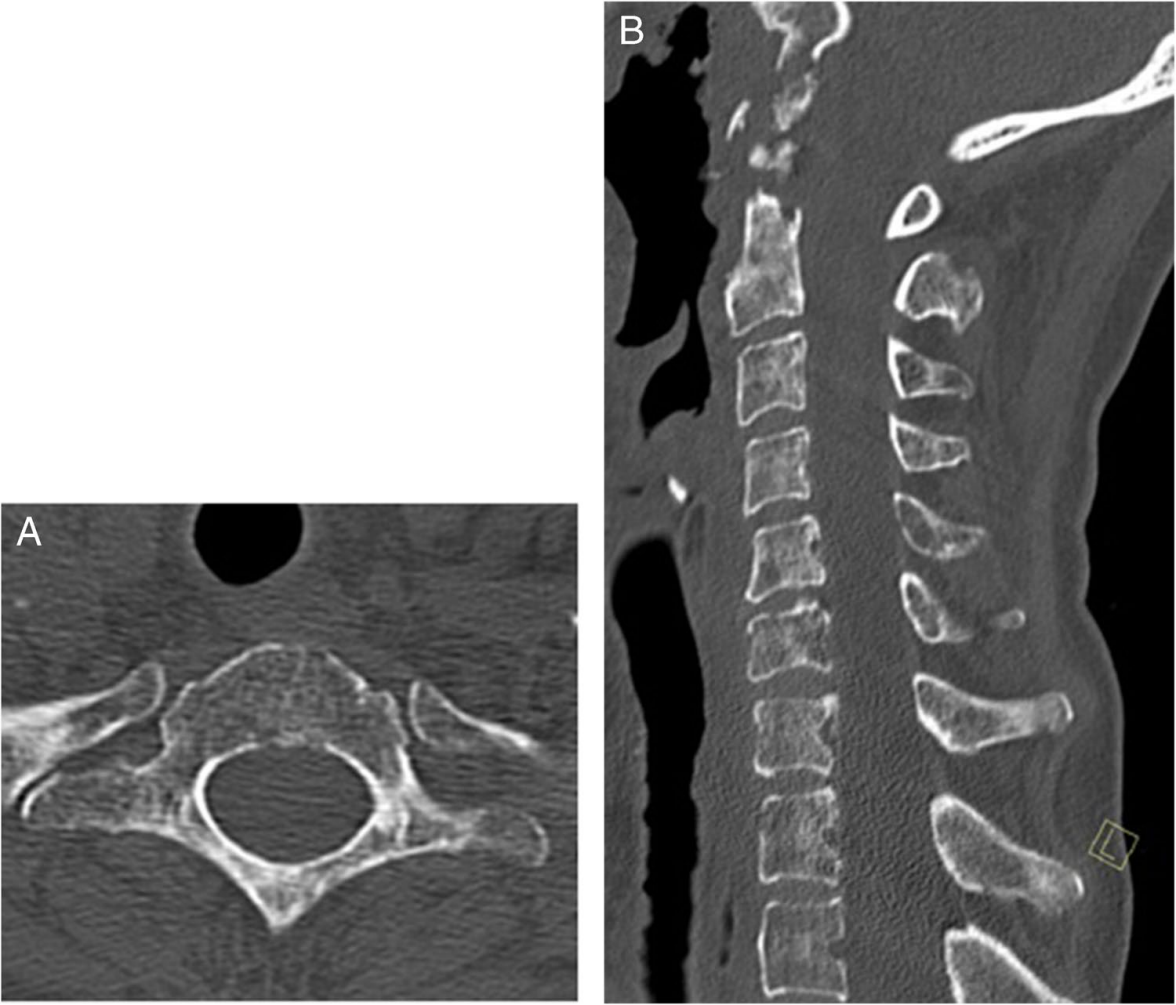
Axial (A; at T1 level) and sagittal midline (B). Noncontrast CT images demonstrate diffuse marrow signal heterogeneity. No focal sclerotic lesions are seen corresponding to the abnormalities seen on previous contrast-enhanced neck CT images (see Fig. 1)
